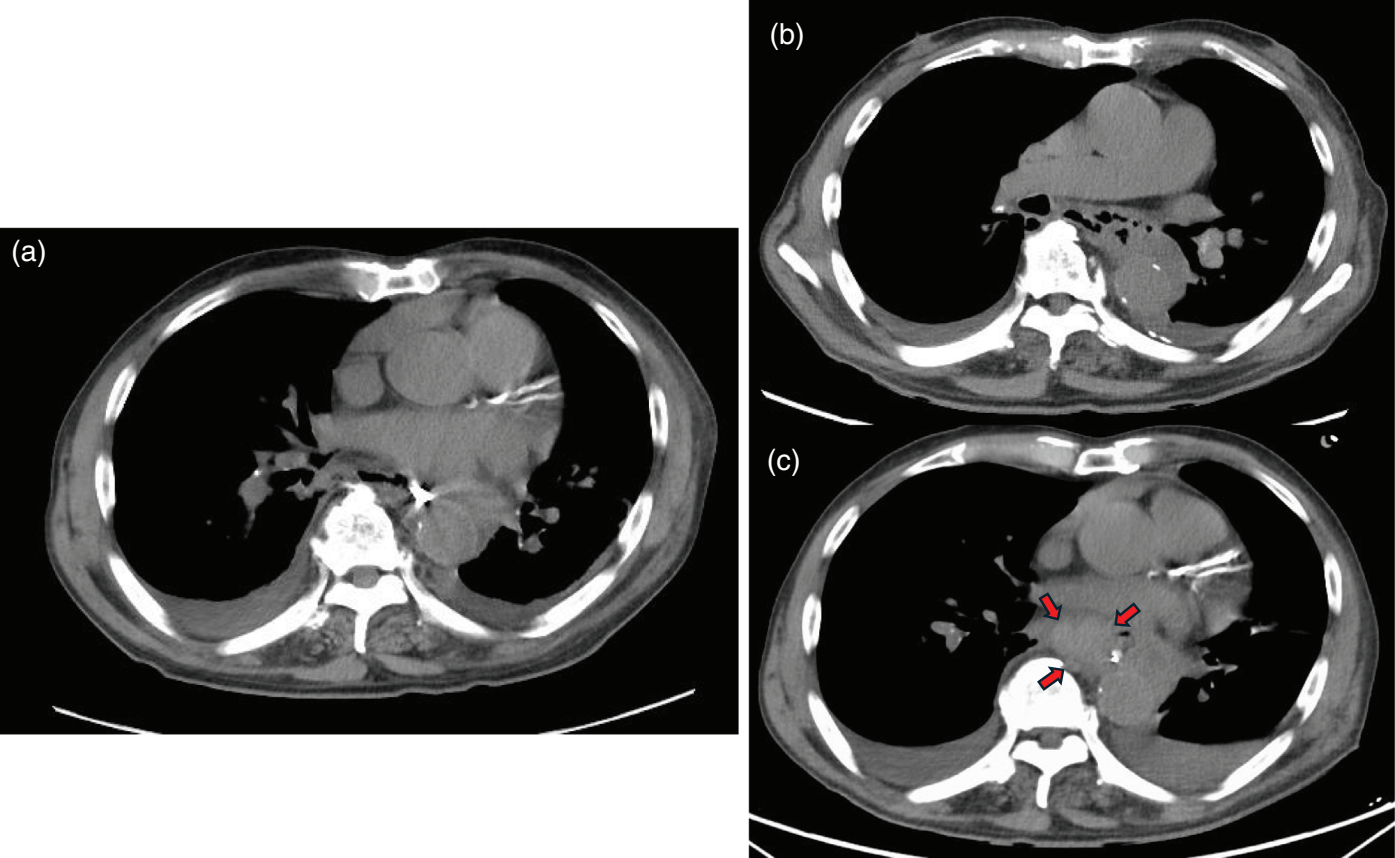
Findings at the time of diagnosis of delayed perforation. Computed tomography showed mediastinal emphysema and bilateral pleural effusion (a). Computed tomography scan just before placement of the water‐expelled drainage tube tube(b). Emergency computed tomography on postoperative day 16 showed the appearance of a hematoma in the mediastinum (red arrow) (c).
